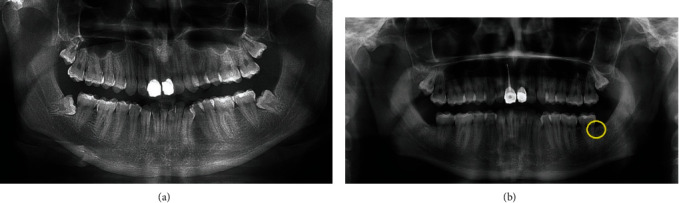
Panoramic radiographs of the patient before surgery (a) and after 6 months: left pocket (yellow circle) still resulted to be deeper than the grafted one (b). No signs of adverse reaction or bone resorption were present at the grafted site.
